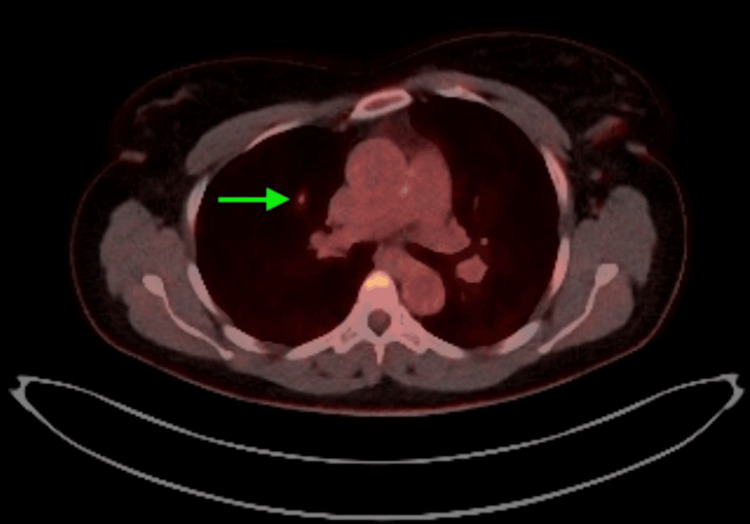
PET-CT scan of a suspicious right upper lobe lesion.
